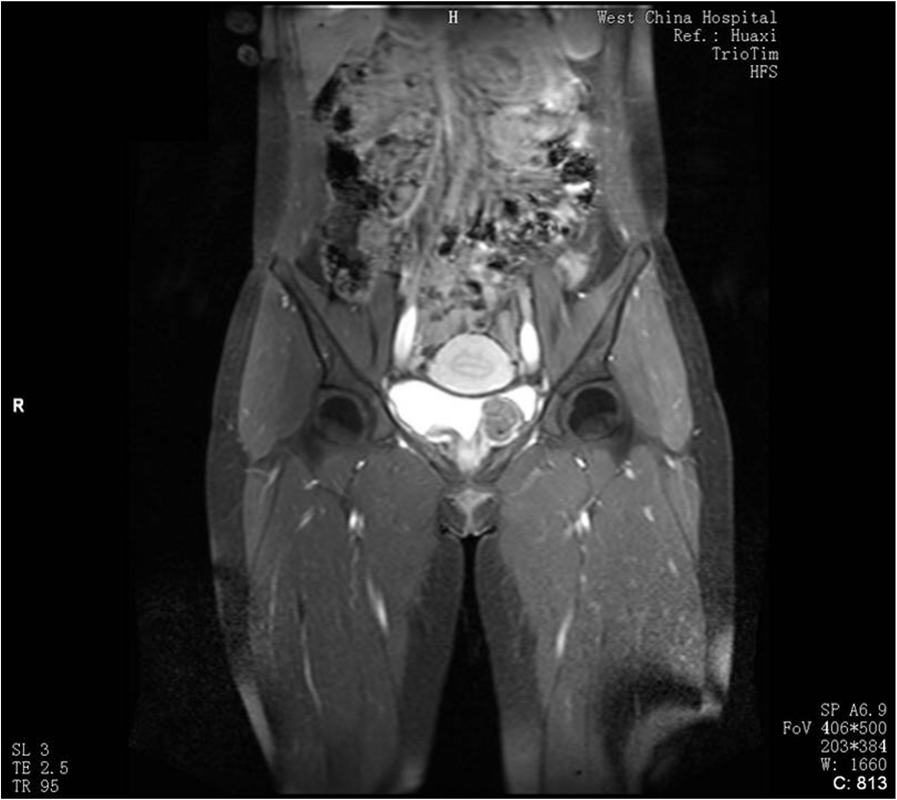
Gd-enhanced coronal T1-weighted MRI showing significantly inhomogeneously enhanced well-defined mass with a wide base.
